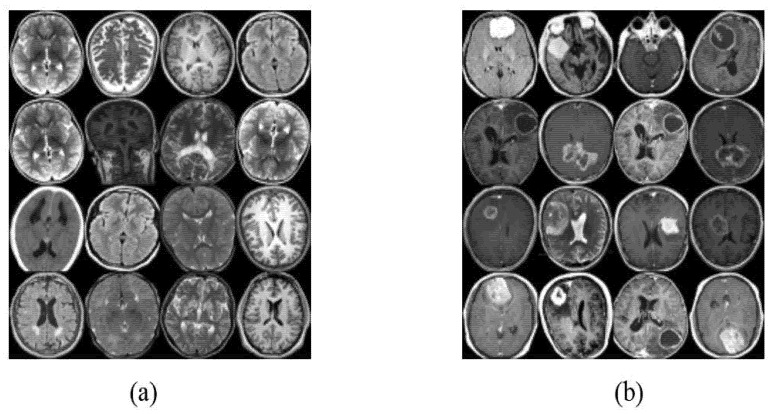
MRI scan images for two classes (a) No tumor samples images, and (b) Tumor sample images.
